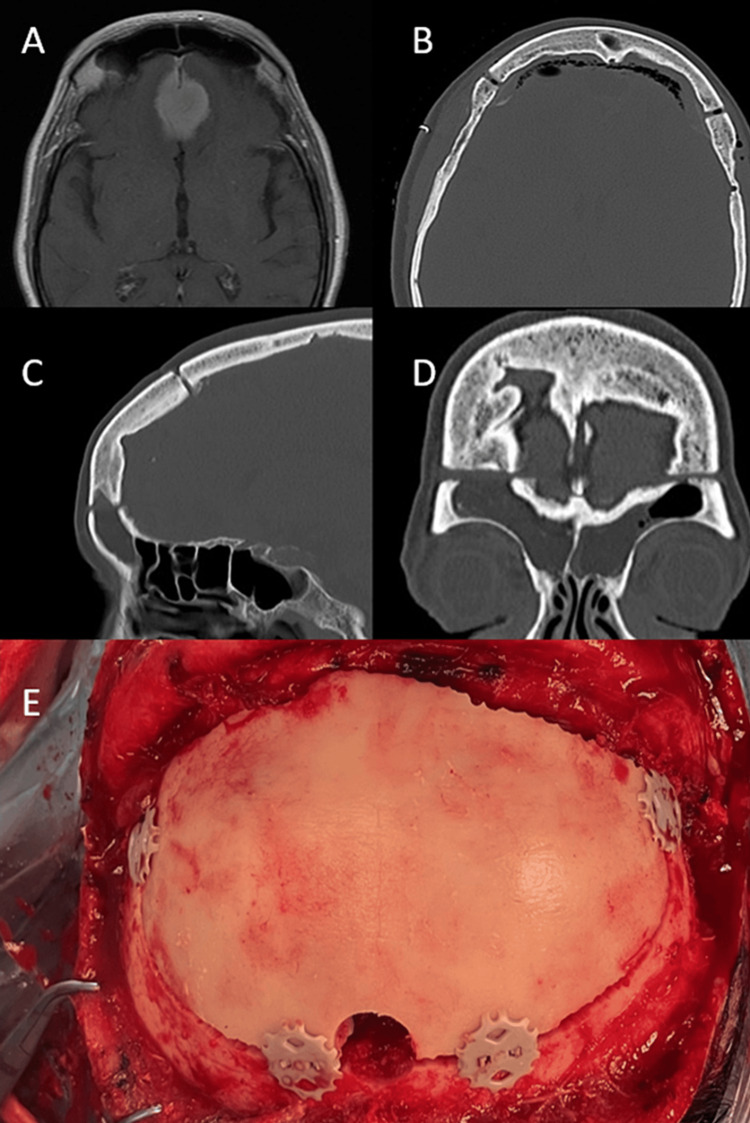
A 72-year-old woman undergoing olfactory groove meningioma resection (A) Pre-operative MRI image: axial view weighted in T1 with gadolinium showing a frontal basal meningioma. (B) Twenty-four-hour post-operative CT scan image: axial view of the bone. (C) Five-month post-operative CT scan image: sagittal reconstruction view of the bone. D) Five-month post-operative CT scan image: coronal reconstruction view of the bone. (E) Surgical view after tumor resection and fixation of the bone flap with Cranial LOOP™.
